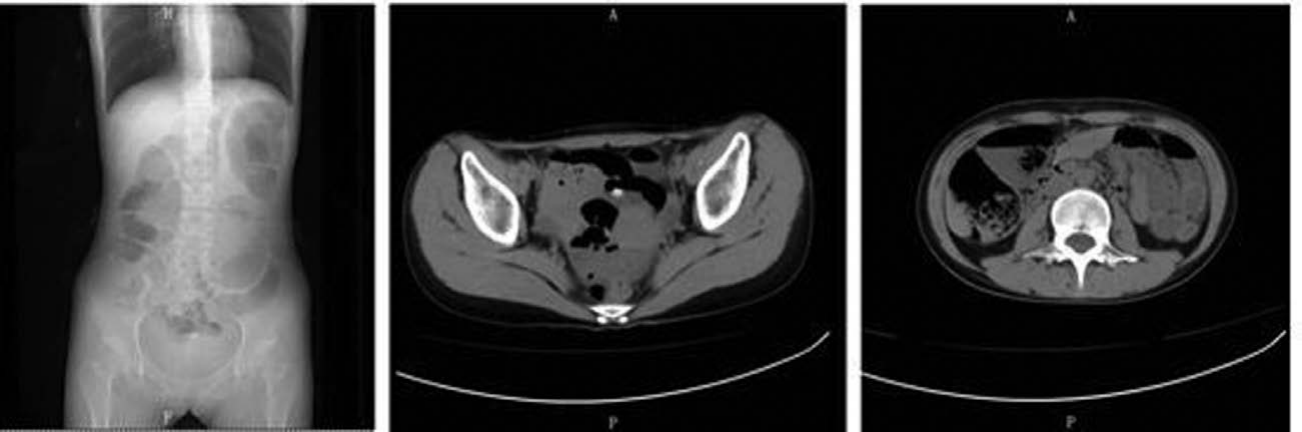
Abdominal CT scan findings: figure shows incomplete intestinal obstruction vs acute pancreatitis exclusion. CT = computerized tomography.
